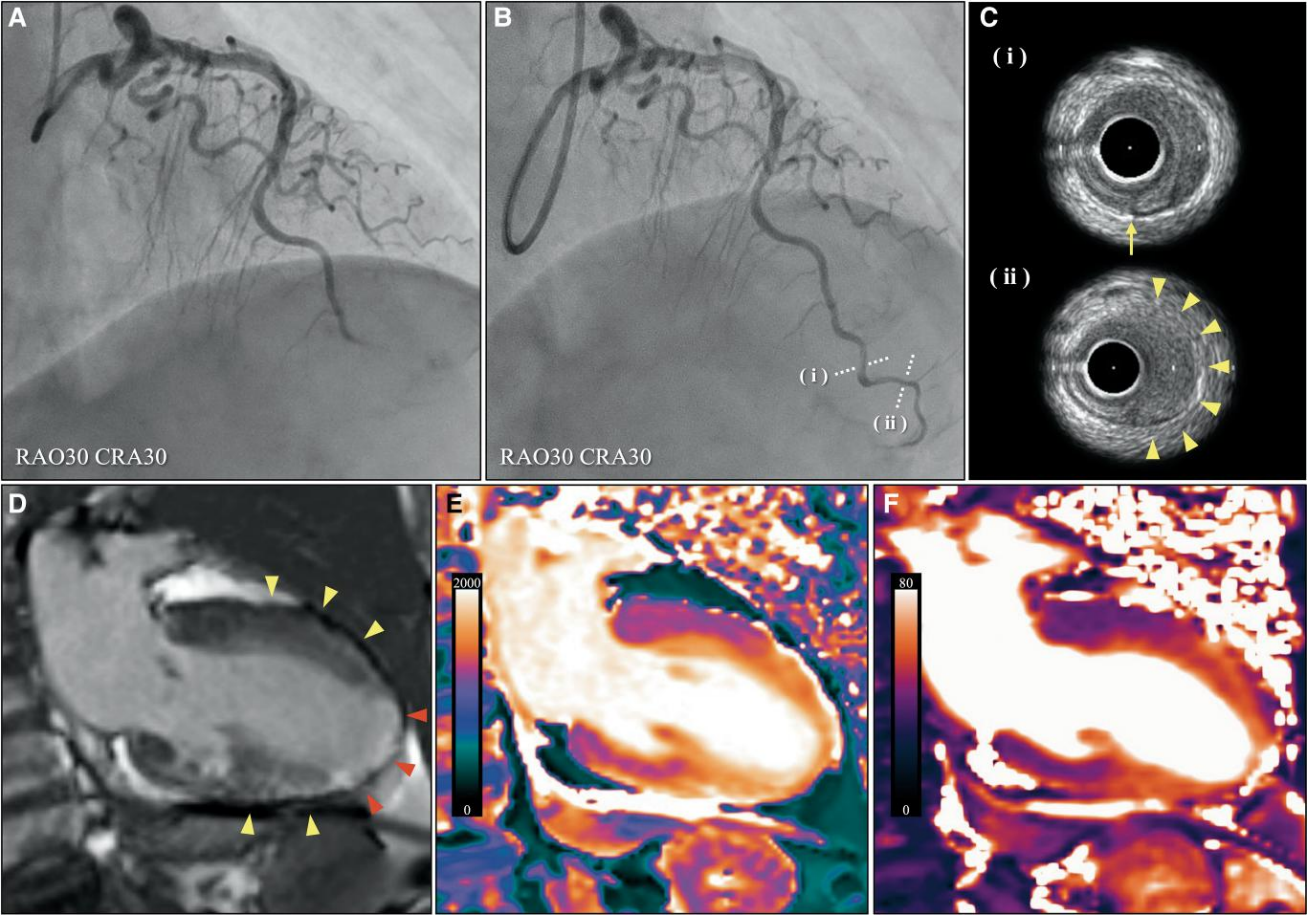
(*A*) Initial and (*B*) post-percutaneous coronary intervention coronary angiography reveals occlusion in the distal left anterior descending artery, which is recanalized through guidewire crossing. (*C*) Intravascular ultrasonography shows an intima tear (arrow) and an intramural haematoma (arrowheads) in each lesion (i, ii) visible on *B*, suggesting spontaneous coronary artery dissection. (*D*) Late gadolinium enhancement of cardiac magnetic resonance imaging demonstrates significant high-signal intensity in the infarcted apex (arrowheads) and mildly high-signal intensity in the transmural layers of the non-infarcted mid-ventricle (arrowheads). (*E*) T1 and (*F*) T2 mapping demonstrate gradually increasing T1 and T2 values from the base to the apex [1227 ms at the base, 1341 ms at the mid, and 1658 ms at the apex in T1 mapping (normal, 1182 ± 24 ms); 40 ms at the base, 51 ms at the mid, and 61 ms at the apex in T2 mapping (normal, 41 ± 1 ms)].
